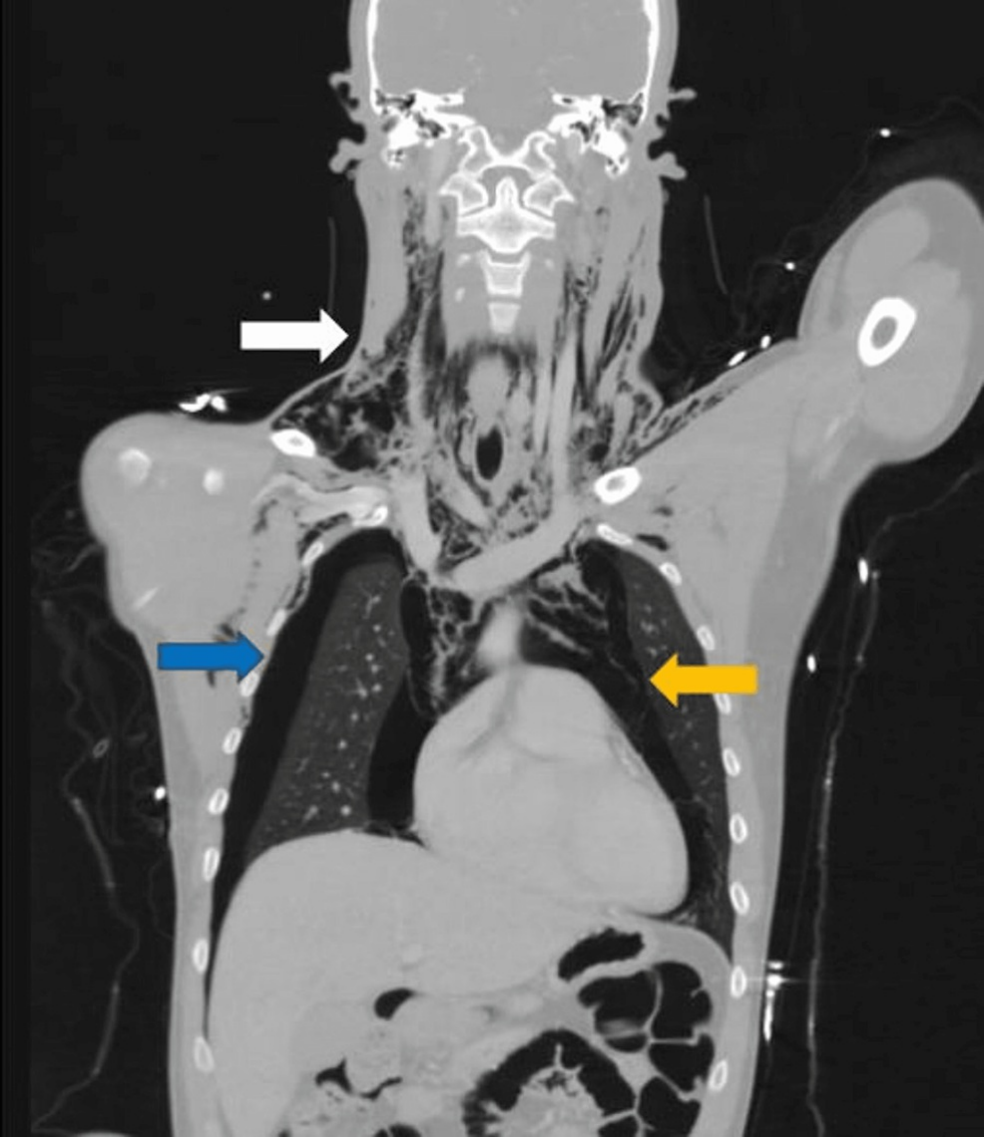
First ICU day thoracic CT: Bilateral pneumothorax, more pronounced in the right lung field (blue arrow), aggravated subcutaneous emphysema (white arrow), and pneumomediastinum (yellow arrow)
